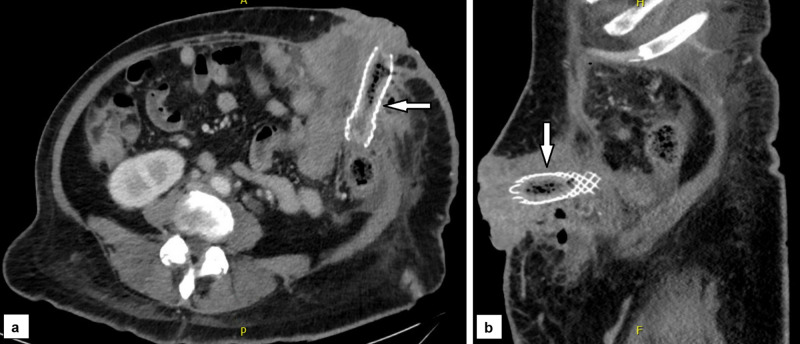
Abdominal CT scan showing the metal stent in place, as seen on axial (a) and sagittal cuts (b)
